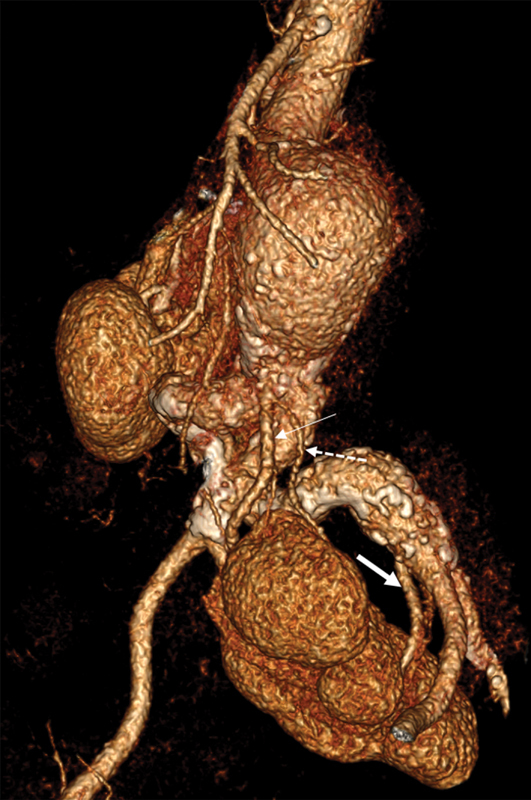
Preoperative computed tomography angiography depicting the left congenital pelvic kidney and the two aberrant renal arteries (RAs) (thick arrow: distal RA, thin arrow: proximal RA, dotted arrow: small branch of the proximal RA).
